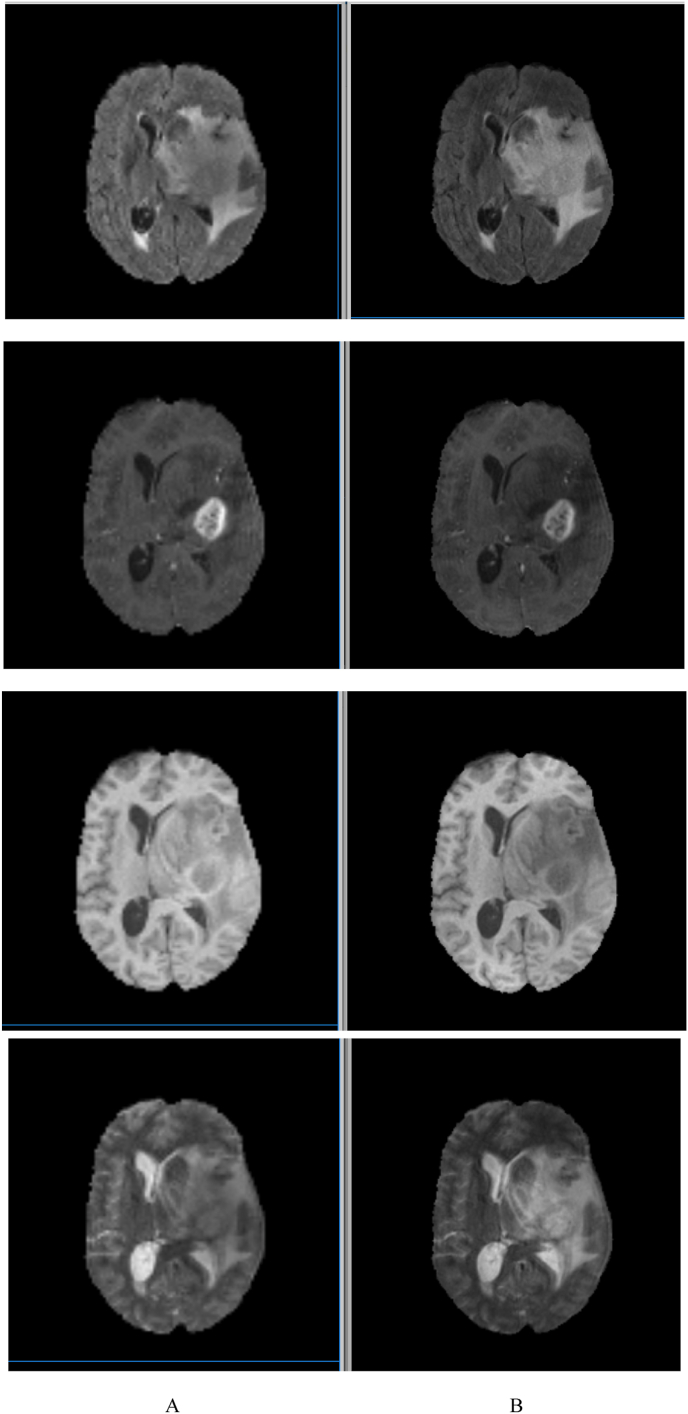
The pre-processed image is displayed alongside the raw image from Brain MRI across four different scan types. A) The processed image, B) The raw MRI image.
